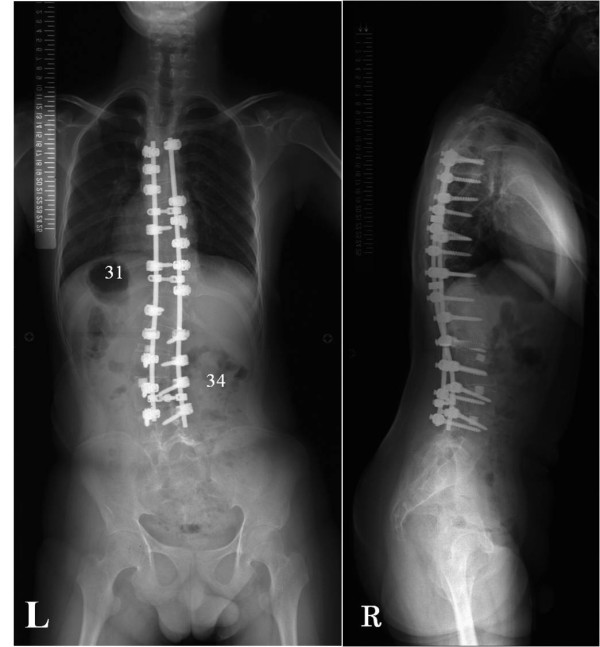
Full-length spine standing radiographs at one year after the second operation. The initial correction is maintained. The Cobb angle from T6 to T12 is 31 degrees and the angle from T12 to L4 is 34 degrees. Left: coronal, Right: sagittal.
